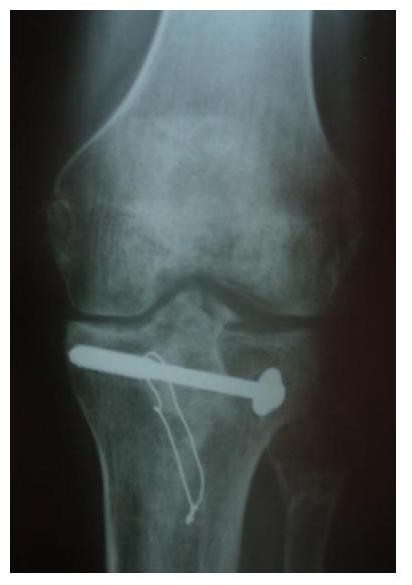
AP x-ray of the same patient after hybrid external fixator removal.
